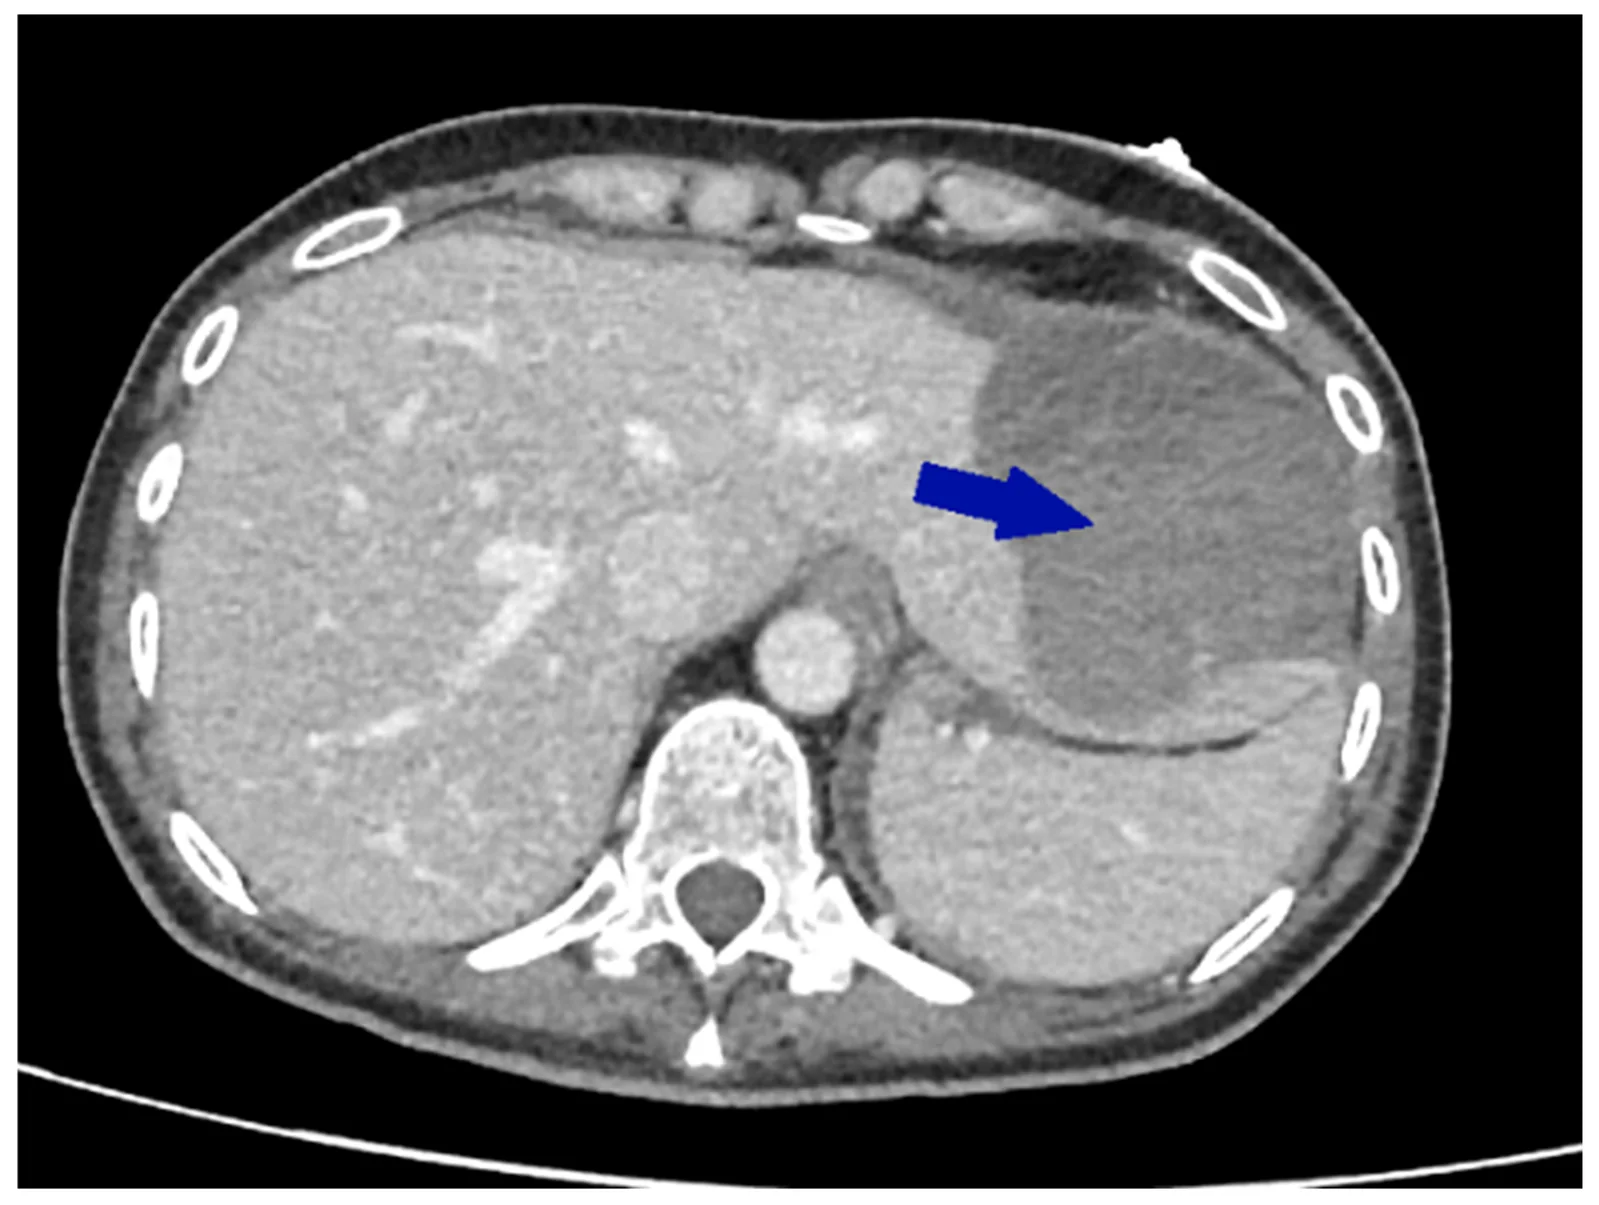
A patient reported abdominal pain while on the ward. The CT scan of the abdomen demonstrated a subcapsular haematoma measuring 99 × 77 × 126 mm in the left hepatic lobe with heterogeneous density, suggesting active haemorrhage—marked with blue arrow. Surgery was performed, and the liver haematoma was evacuated.
